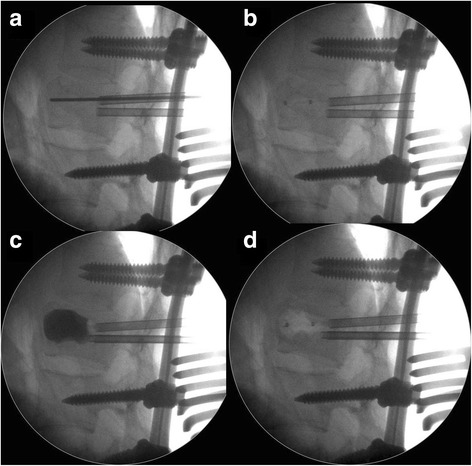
Radiographic image of kyphoplasty technique. Kyphoplasty was performed using a cannula and an expander, which were inserted into the pedicle, as well as a drill, which was inserted through the cannula. A needle pipe and pin was placed parallel to the superior endplate in the lateral view (a). The balloon was slowly inflated with initial bulk pressure (b and c). The volume of the balloon was carefully controlled to restore the fractured vertebra until adequate kyphotic angle reduction was obtained or the inflation pressure reached 220 psi [19]. The operator should record the amount of injected fluid to predict the cement volume. The balloon was deflated and withdrawn (d), and the created cavity was filled with cement [19]
